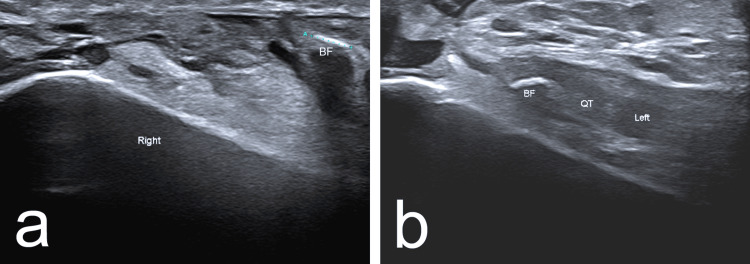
Longitudinal musculoskeletal ultrasound images of the suprapatellar region. (a) Right knee and (b) left knee demonstrating complete disruption of the quadriceps tendons with loss of normal fibrillar continuity and associated hematoma within the defect. Small, avulsed bone fragments are also visible adjacent to the superior patellar poles. BF: bone fragment; QT: quadriceps tendon
